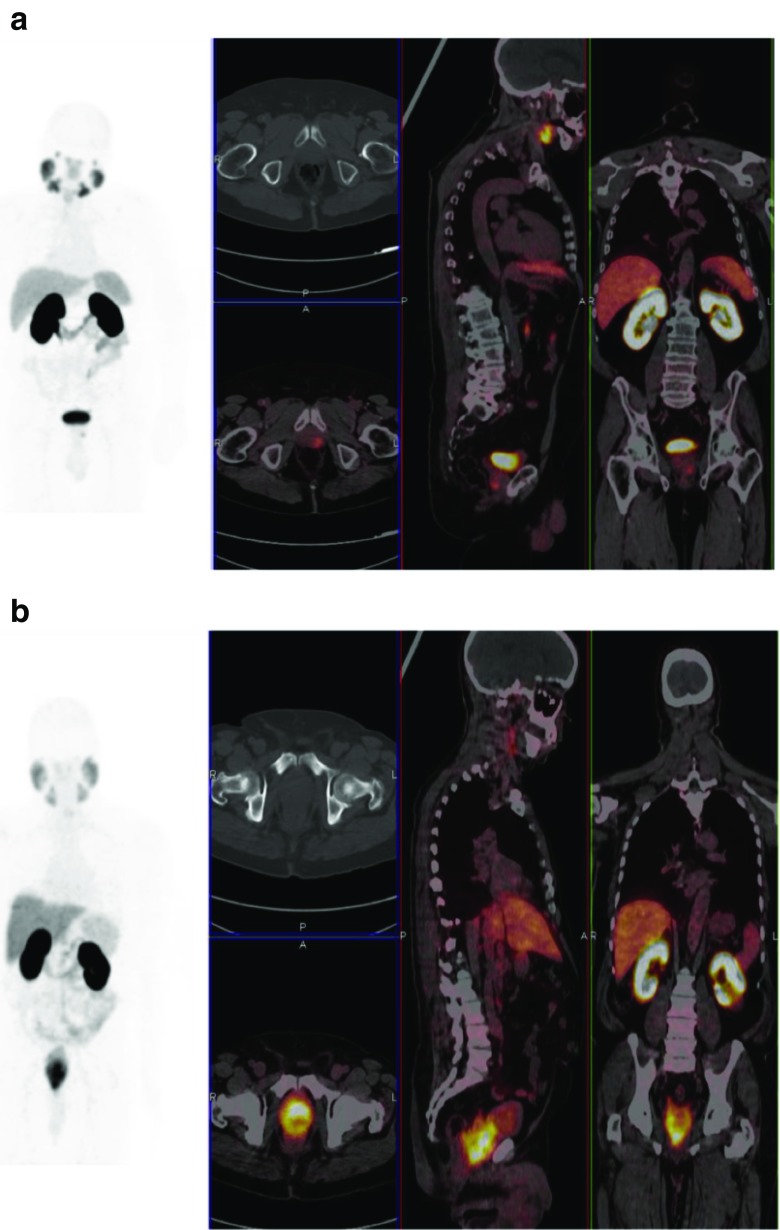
Comparison of SUV max values of primary prostate carcinoma for two patients with the same Gleason scores (4 + 5) but different races (A) corresponds to a 70-year-old white man, maximum-intensity-projection PET, axial, coronal and sagittal fused PET/CT demonstrated SUVmax of 6.3 in the primary prostate cancer with a PSA of 10.5 ng/mL. (B) corresponds to a 69-year-old black man, maximum-intensity-projection PET, axial, coronal and sagittal fused PET/CT demonstrated SUVmax of 29.2 in the primary prostate cancer with a PSA of 38.71 ng/mL
